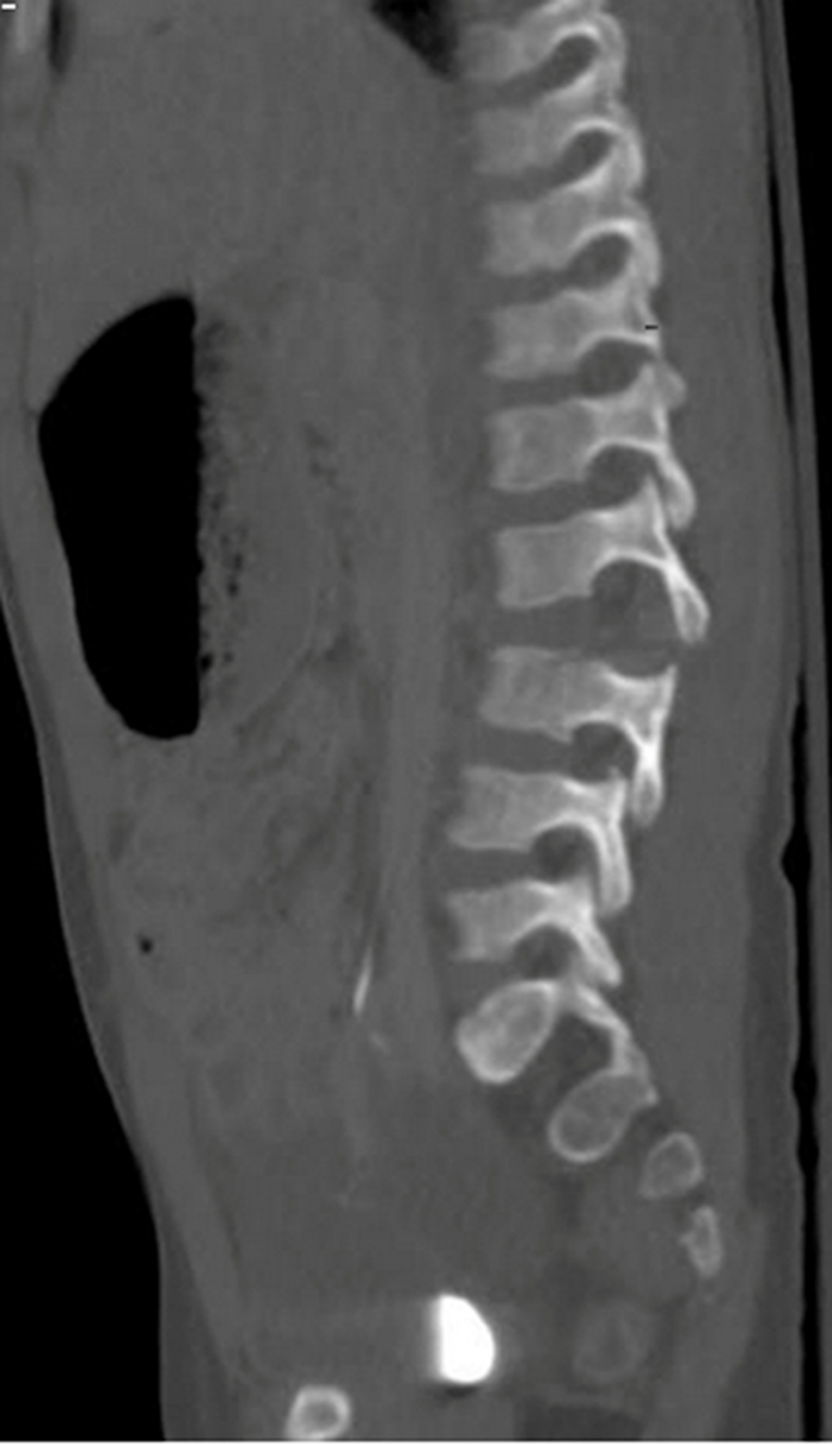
Sagittal CT scan with contrast of the lumbar spine right of midline. There is angulation at the L2-3 level with widening of the posterior intervertebral disc space and the spinous processes, findings consistent with ligamentous injury. Fracture was not detected in this series.
